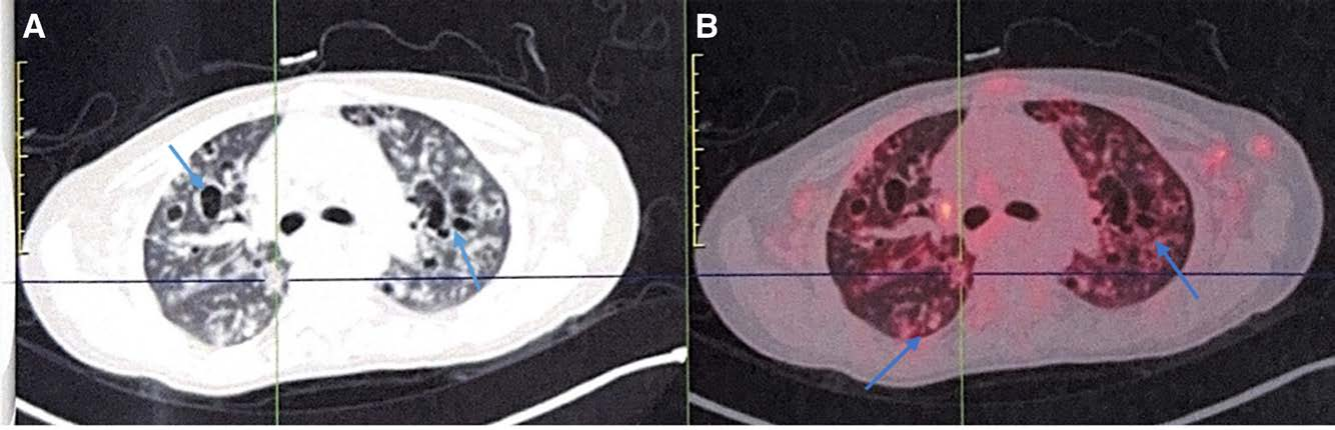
Examination of 18F-fluorodeoxyglucose/positron emission tomography (18F-FDG/PET-CT). (A) Multiple pulmonary nodules (blue arrow); (B) varying degrees of increased FDG metabolism.
